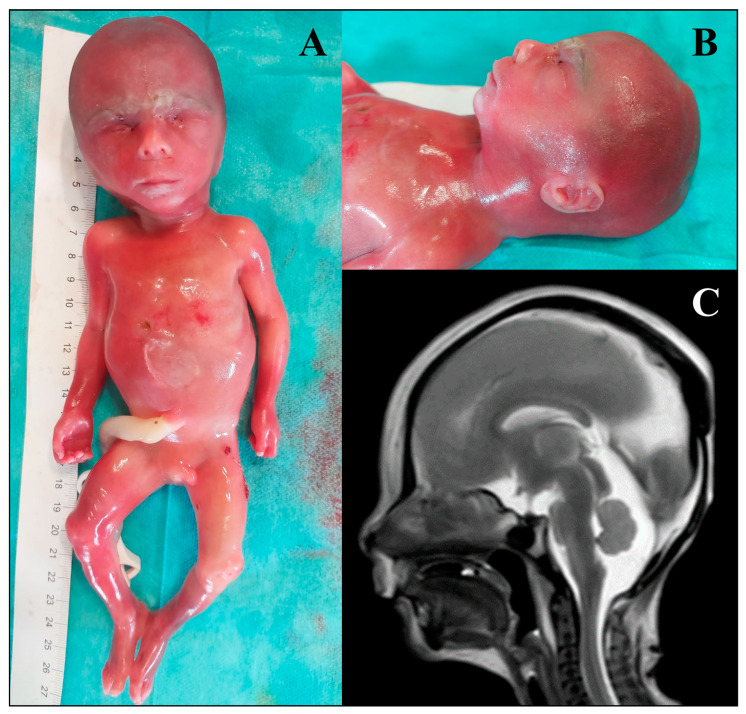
Phenotypic features of the foetus harbouring a heterozygous de novo variant in the *ZNF292* gene. (A) Overall foetal appearance: total body length: 26 cm; crown–rump length: 16 cm; head circumference: 19.2 cm; thoracic circumference: 16.8 cm; right foot sandal gap; normal male genitalia. (B) Left facial profile: mild microretrognathia. (C) foetal brain MRI: hypoplasia of the corpus callosum.
